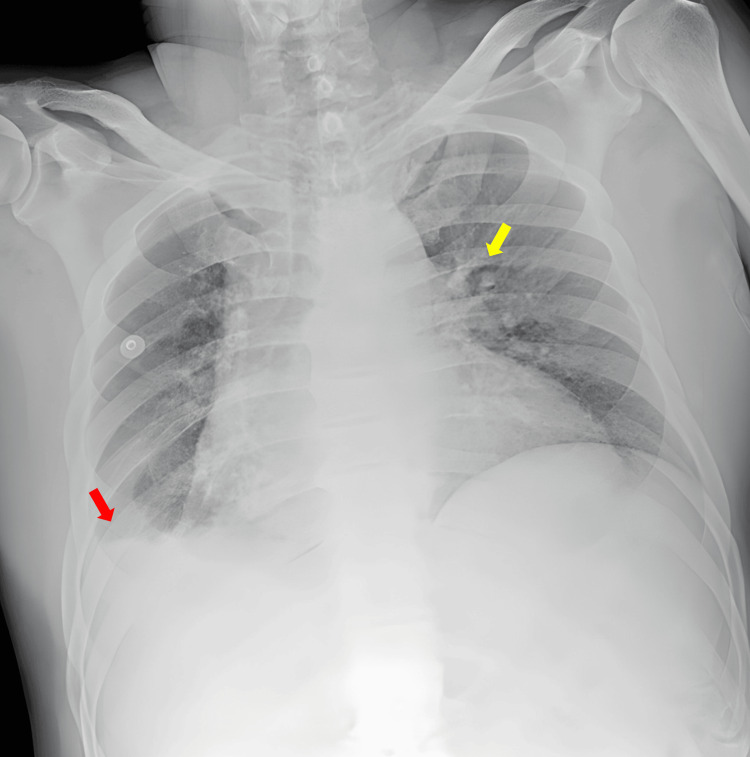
Chest radiograph showing pulmonary vascular congestion and right-sided pleural effusion (Case 1) Chest radiograph demonstrating pulmonary vascular congestion with cephalization of flow (yellow arrow) and a small right-sided pleural effusion with blunting of the right costophrenic angle (red arrow). Findings are consistent with cardiopulmonary involvement in the setting of thyroid storm.
